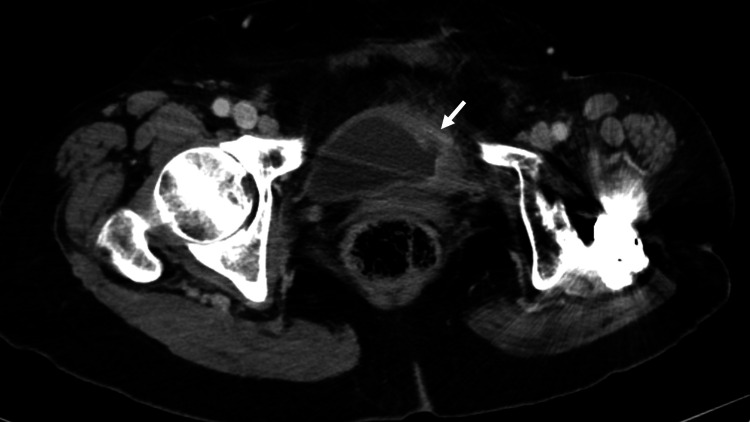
Computed tomography imaging showing an invasive bladder tumor extending from the bladder dome to the left lateral wall (arrow)
